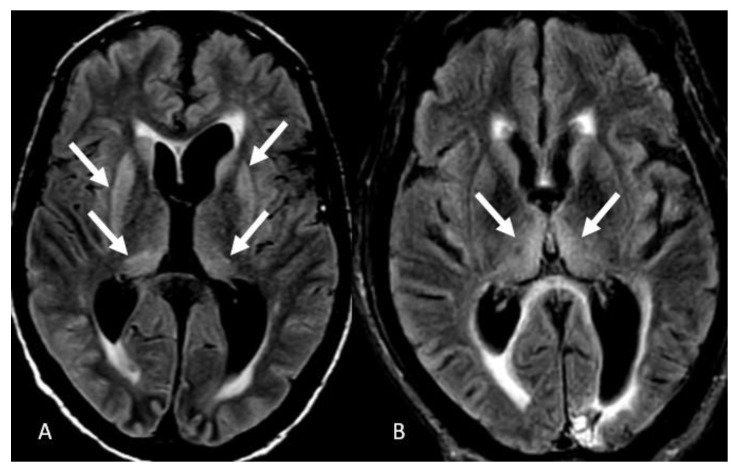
Basal ganglia and thalami involvement. (A) Axial FLAIR images show bilateral basal ganglia and thalami FLAIR hyperintensities. (B) Bilateral thalami FLAIR hyperintensities. Image has been windowed manually to improve the visibility of signal abnormality.
